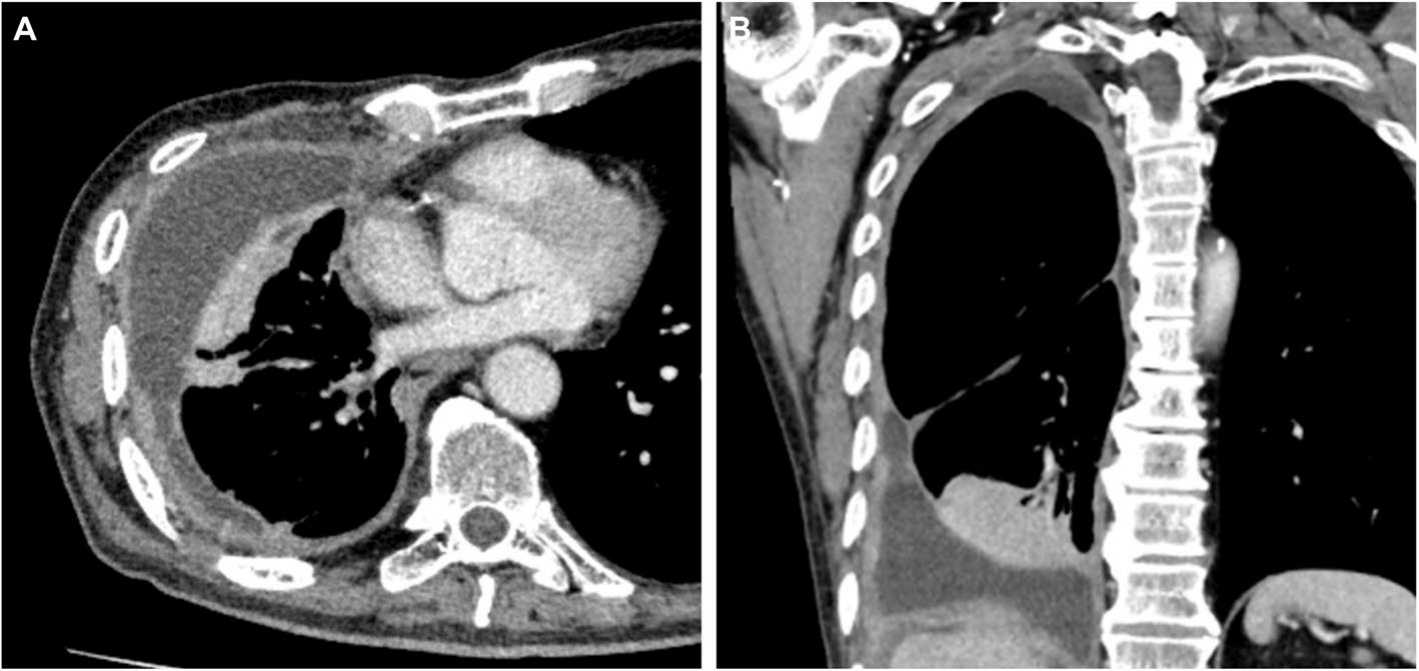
Preoperative chest computed tomography after chemotherapy. A, B Contrast-enhanced chest computed tomography reveals right pleural effusion with thickening of the pleura. Tumor invasion into the lung, diaphragm, and pericardium is suspected
